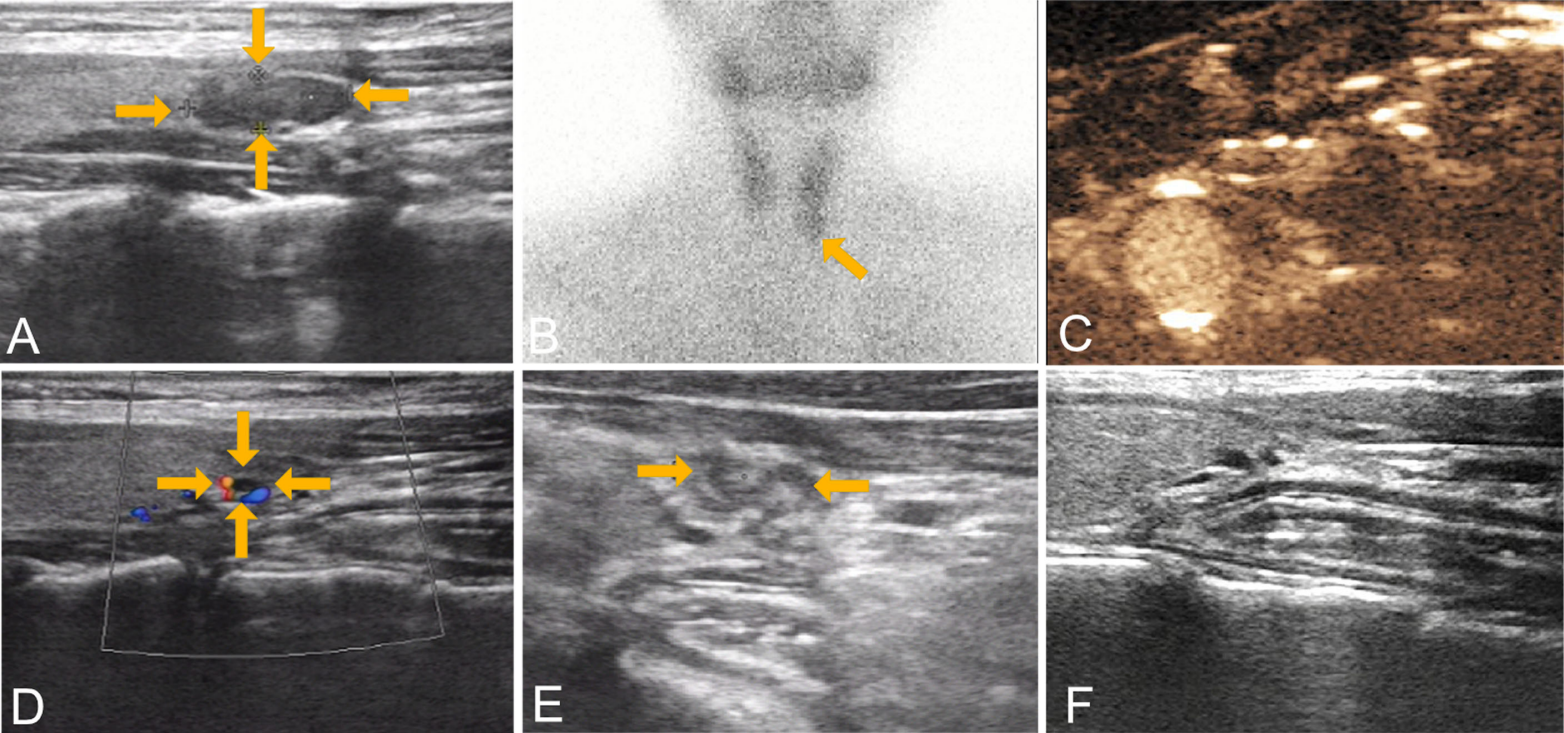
Images from a 30-year-old female with a left lower parathyroid adenoma (13.0 mm × 4.4 mm × 1.3 mm) who underwent microwave ablation therapy. (A) preoperative gray scale ultrasound; (B) preoperative radionuclide scanning; (C) immediate contrast-enhanced ultrasound after ablation; ultrasound examination at three months (D), six months (E) and twelve months (F). Shown by ultrasound, the volume reduction rates were 92%, 98% and 100%, respectively. PHPT, primary hyperparathyroidism; MWA, microwave ablation.
